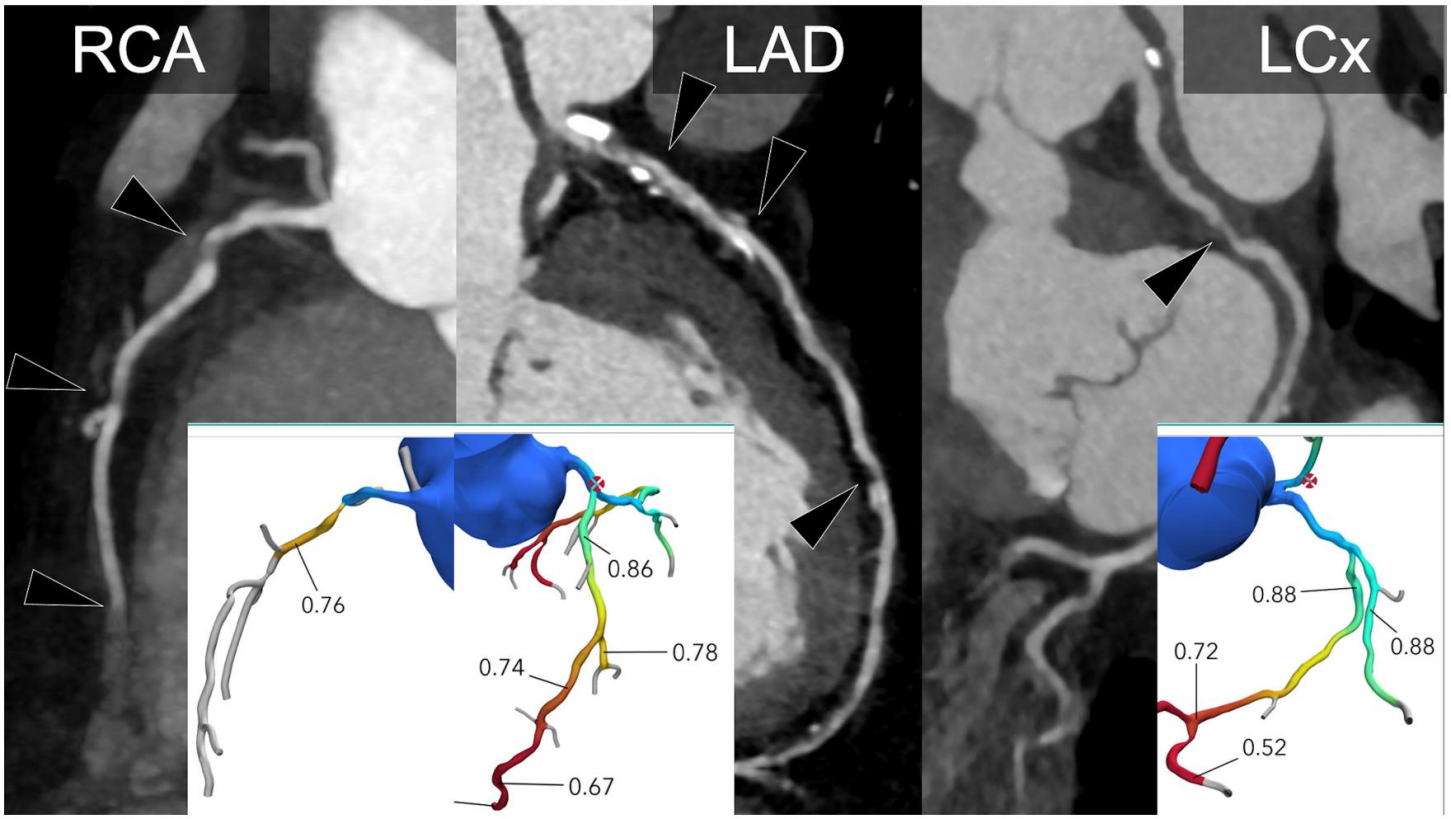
A female patient in her 50s presenting with typical chest pain. Coronary computed tomography angiography (CCTA) reveals multifocal non-calcified plaque causing >70% diameter stenosis in the RCA, multifocal moderate (50%-69%) stenosis in the LAD, and moderate (50%-69%) stenosis in the mid LCx (arrows). Computed tomography-derived fractional flow reserve (CT-FFR) shows the plaque is limiting the blood flow in the RCA, mid LAD, and distal LCx. Findings were confirmed on subsequent ICA, with the patient undergoing coronary artery bypass, with grafts to the LAD, OM1, and left-posterior descending artery. Abbreviations: LAD = left anterior descending artery; LCx = left circumflex artery; RCA = right coronary artery.
